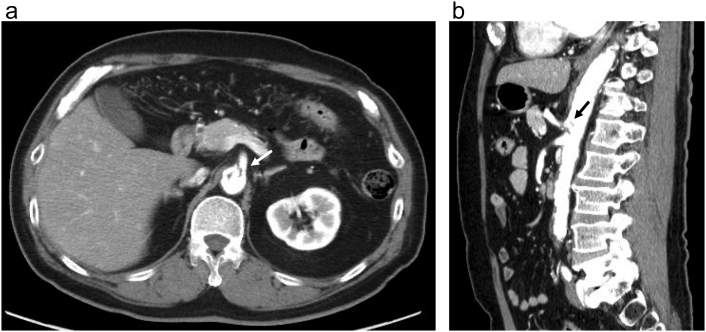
Axial (a) and sagittal (b) contrast-enhanced computed tomography images acquired 3 months after the injury showing an intimal tear shrinkage (arrow).
